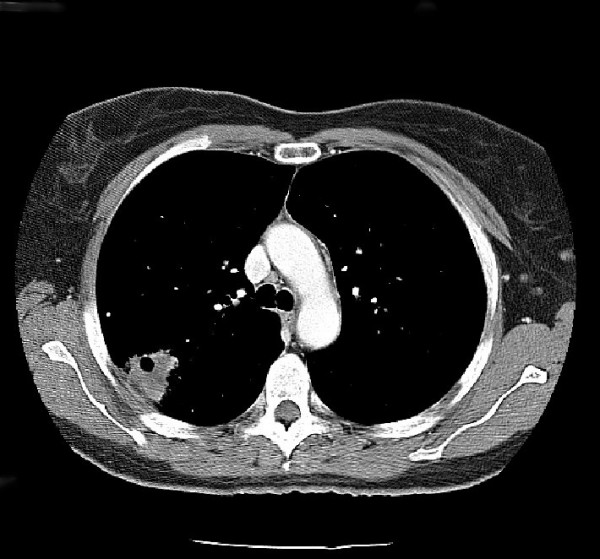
Contrast-enhanced CT scan of the chest for patient #1. A large cavitating lesion was identified in the right upper lobe with pleural involvement and likely extension into the chest wall. In addition, right hilar lymphadenopathy was suspected. This was the primary malignant lesion in this patient.
